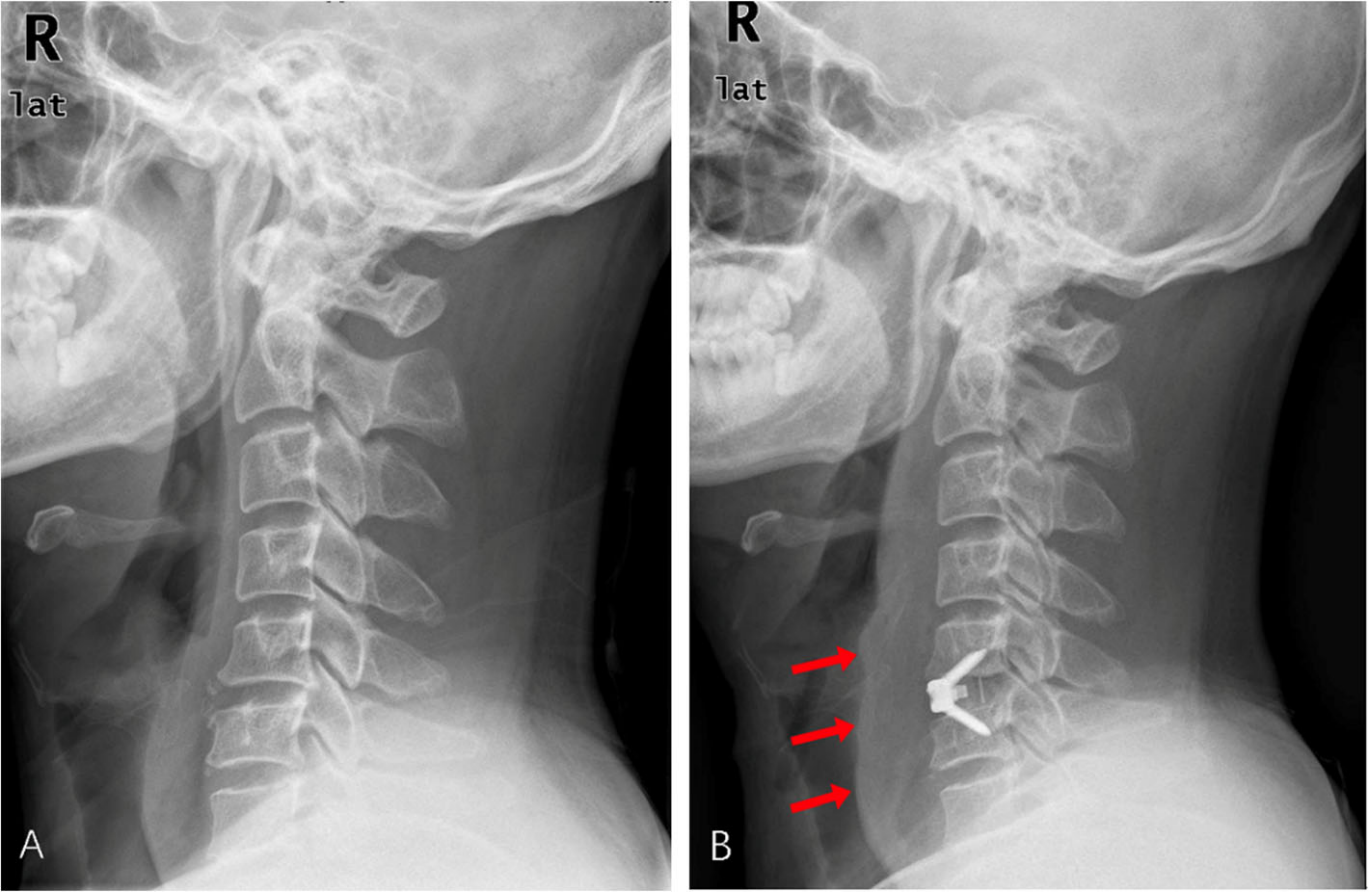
The preoperative (a) and postoperative (b) lateral X-rays of a 37-year-old male patient who was diagnosed with cervical spondylotic myelopathy and underwent C5/6 ACDF with Zero-P. The PSTS was 6.3 mm. The Bazaz grading system showed severe postoperative dysphagia. The X-rays showed obvious PSTS (red arrows). PSTS: Prevertebral soft-tissue swelling
